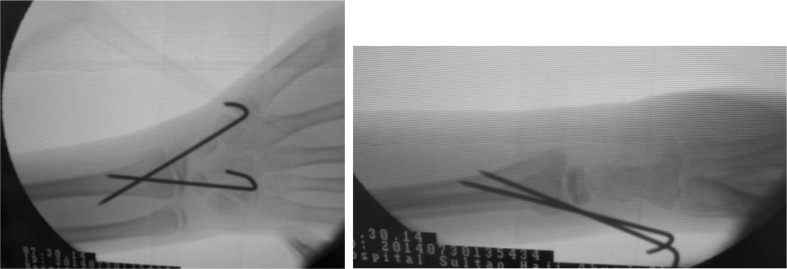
Radiograph under image intensifier guidance for distal end radius after reduction and K-wire insertion.
